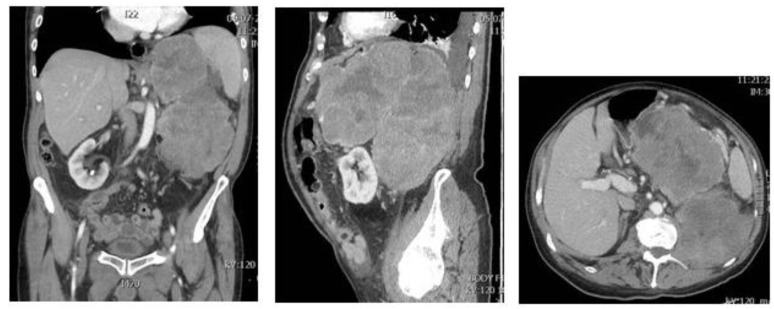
MRI pictures of a big retroperitoneal tumor in an 82-years-old male patient.
